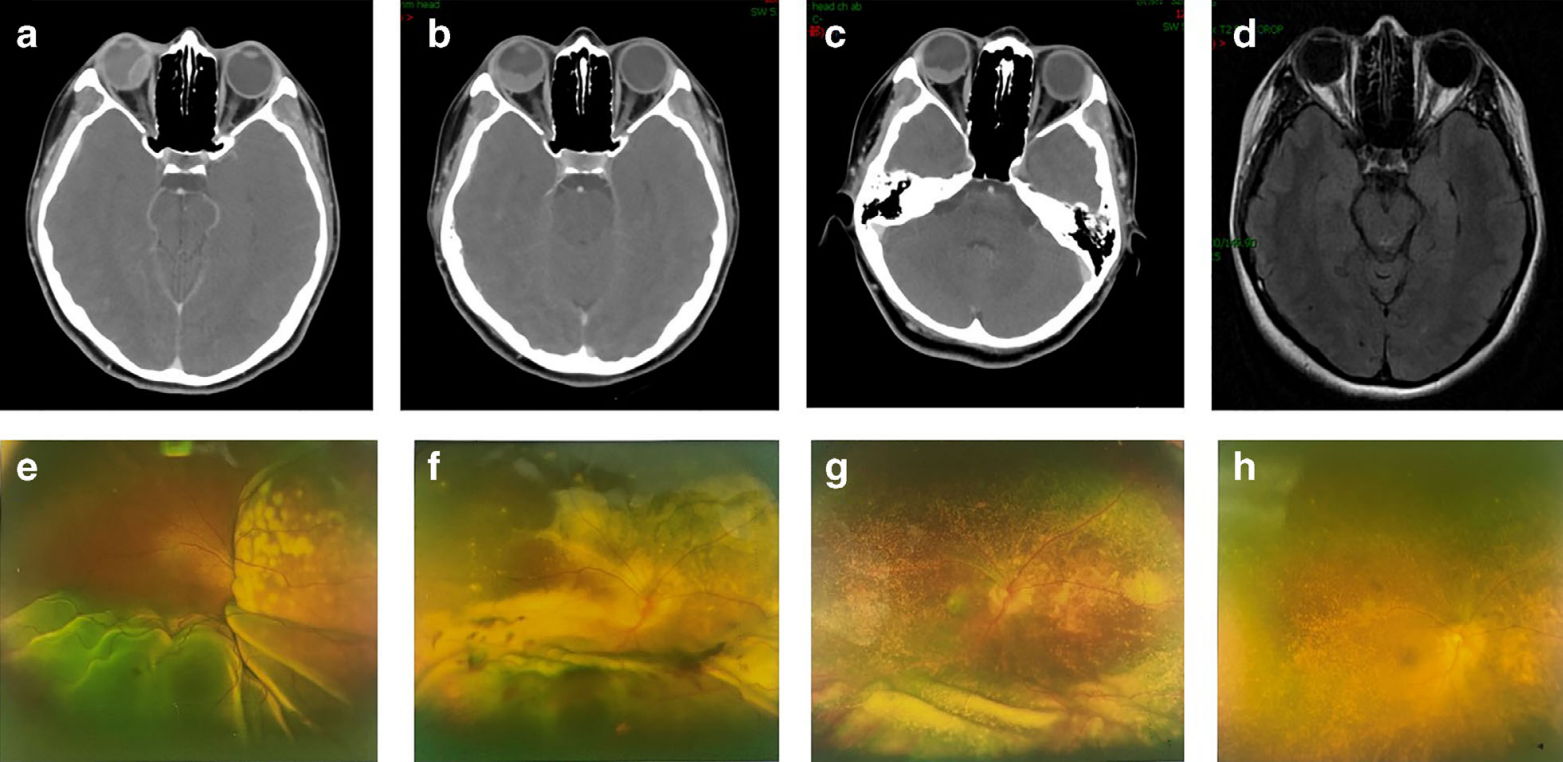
The changes in the metastatic lesion in the right eye during treatment. (a–c) Enhanced computed tomography (CT); (d) brain magnetic resonance imaging (MRI); (e–g) color photography. (a,e) Before all treatments; (b,f) after two cycles of pemetrexed/carboplatin, and transpupillary thermotherapy (TTT); (c,g) after four cycles of pemetrexed/carboplatin, TTT, one dose of ranibizumab; and (d,h) after six cycles of pemetrexed/carboplatin, and three doses of ranibizumab.
